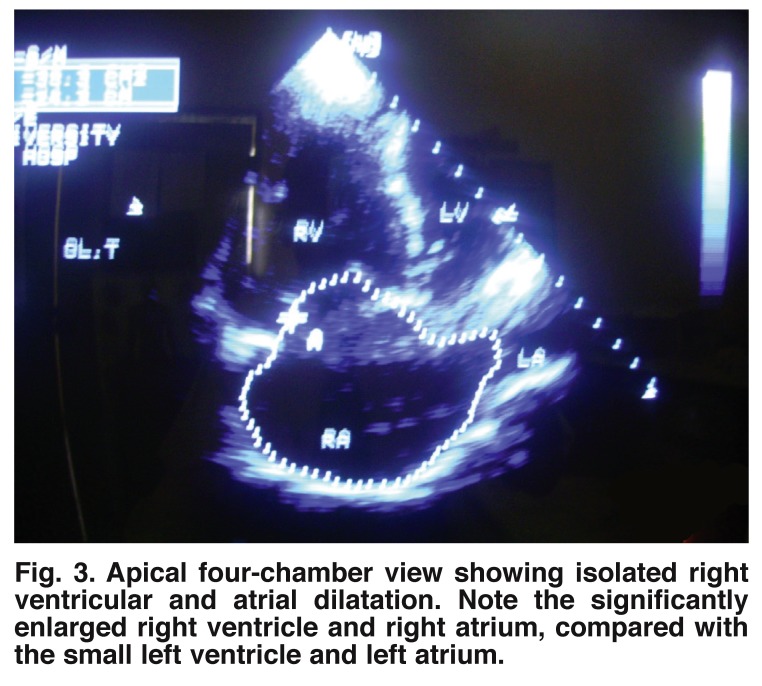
Apical four-chamber view showing isolated right ventricular and atrial dilatation. Note the significantly enlarged right ventricle and right atrium, compared with the small left ventricle and left atrium.
